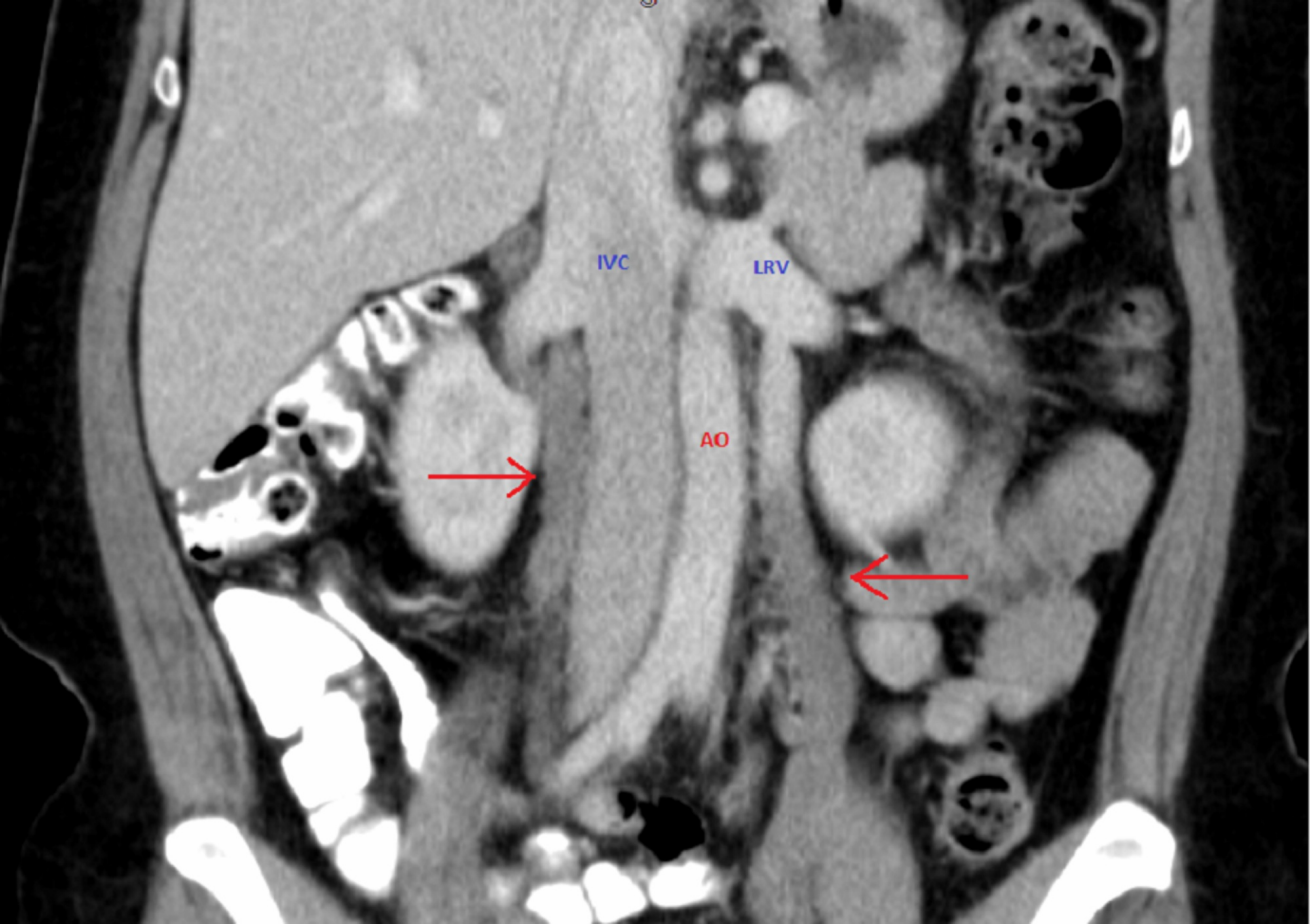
CT of the abdomen (coronal section) showing bilateral OVT as indicated by the red arrows CT: computed tomography; OVT: ovarian vein thrombosis; AO: abdominal aorta; IVC: inferior vena cava; LRV: left renal vein
